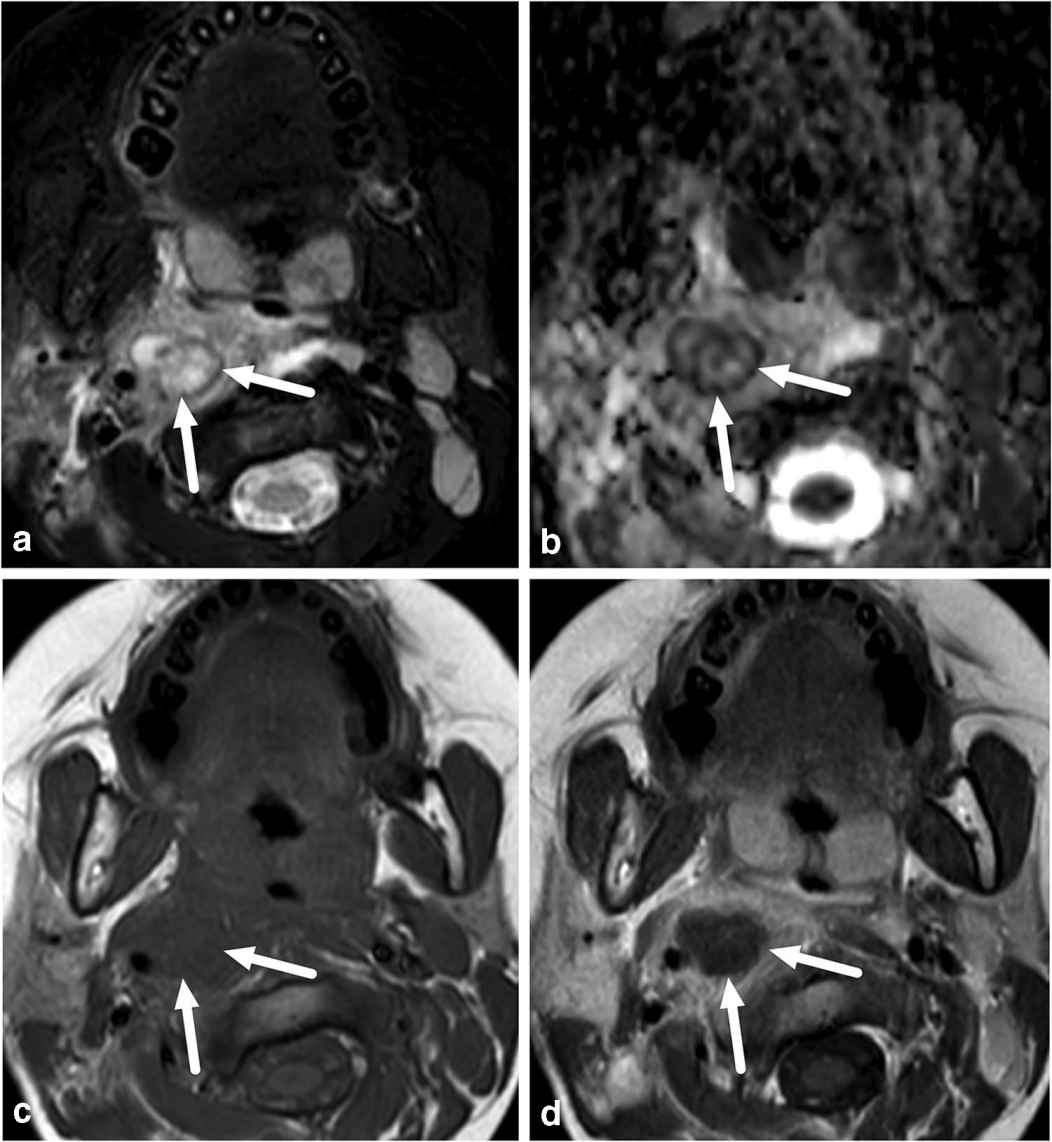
Retropharyngeal suppurative lymphadenitis in a 7-year-old girl with a sore throat and streptococcal tonsilitis. a–d Axial images show a focal mass in the right lateral retropharyngeal space (*arrows*) with an intermediate T2-signal (a), low apparent diffusion coefficient consistent with restricted diffusion (b) and no central contrast enhancement (c T1-weighted, before contrast; d T1-weighted after contrast), consistent with suppurative lymphadenitis. Purulence was found during surgery
